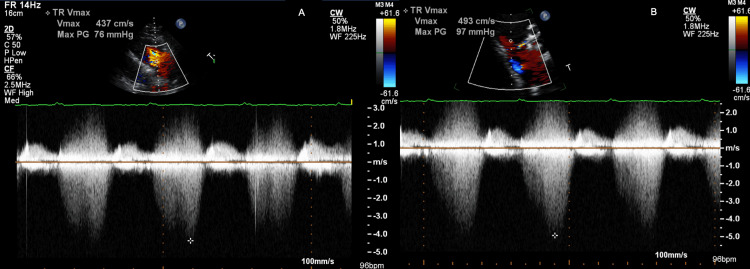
TTE image of RV inflow view. Panel A with a Doppler waveform across the tricuspid valve with a Vmax of 4.37 m/s originally described as "tricuspid regurgitation." Evidence of a double envelope across the tricuspid valve secondary to the VSD seen at the white star. Panel B with a double dagger envelope, Vmax of 4.93 m/s, occurring in mid-systole suggestive of a VSD. TTE: transthoracic echocardiogram; RV: right ventricle; VSD: ventricular septal defect
